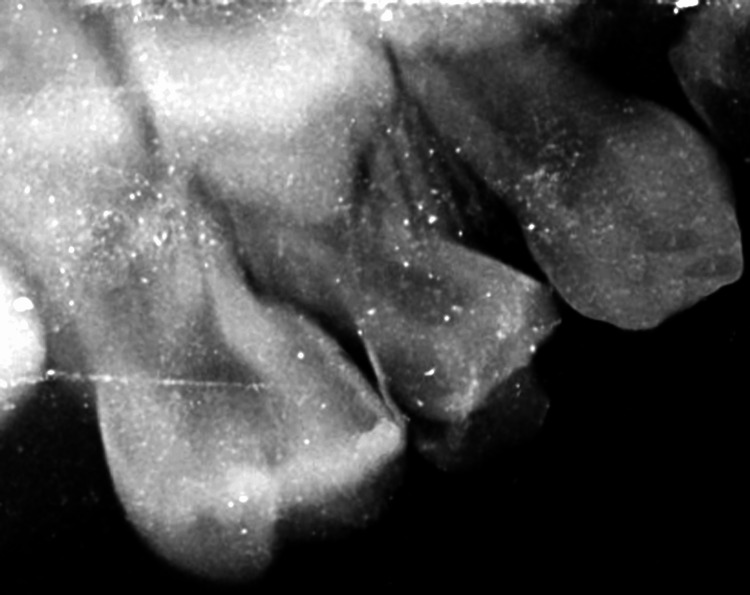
Pre-operative radiograph showing grossly carious 54.
